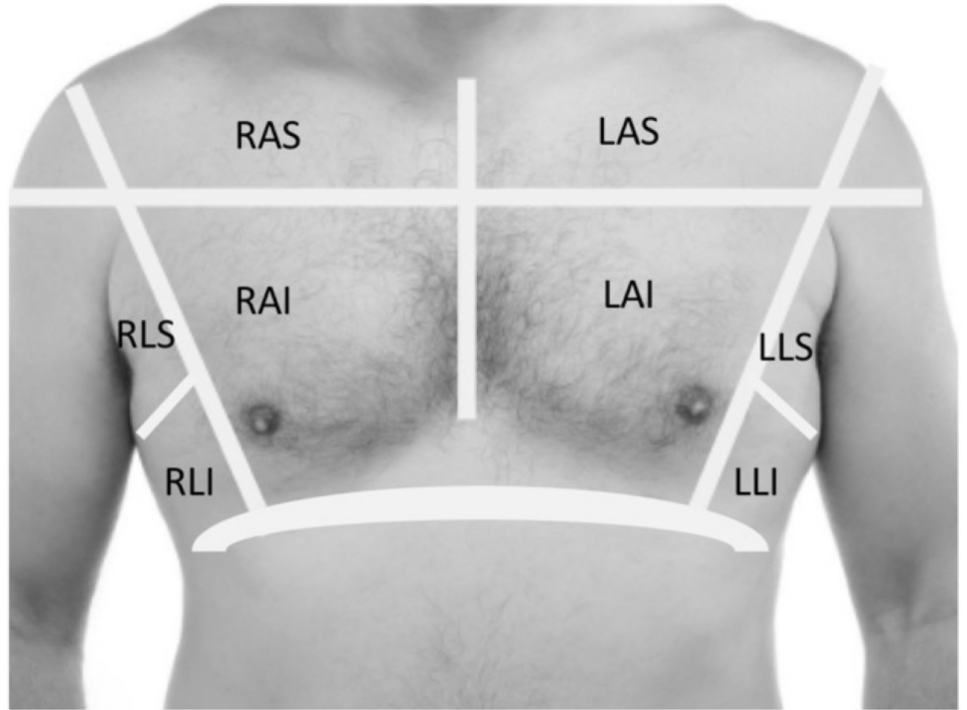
Location of ultrasound interrogation of thorax for B-line presence and quantity: right anterior superior (RAS), right anterior inferior (RAI), right lateral superior (RLS), right lateral inferior (RLI), left anterior superior (LAS), left anterior inferior (LAI), left lateral superior (LLS) and left lateral inferior (LLI)
